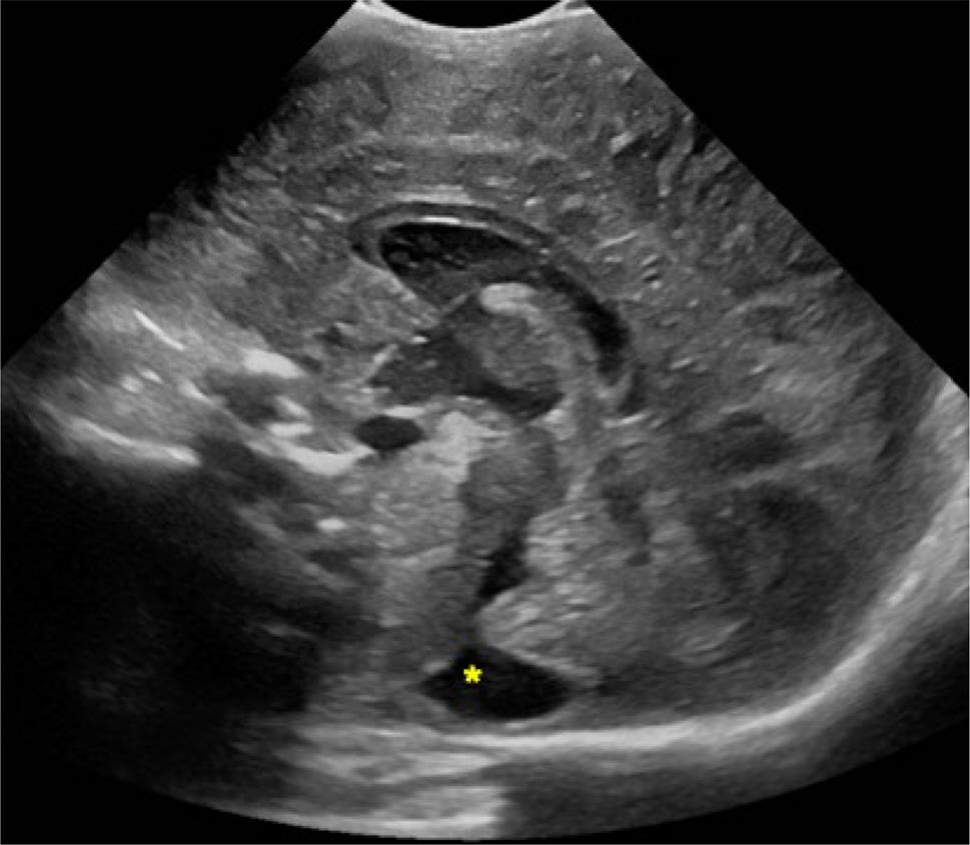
Screening cerebral ultrasound of a premature female born at 32 weeks and 6 days of gestation showing on sagittal view well-defined anechoic space located in the posterior cranial fossa (yellow asterisk). This finding was suggestive of mega cisterna magna (common anatomical variant)
